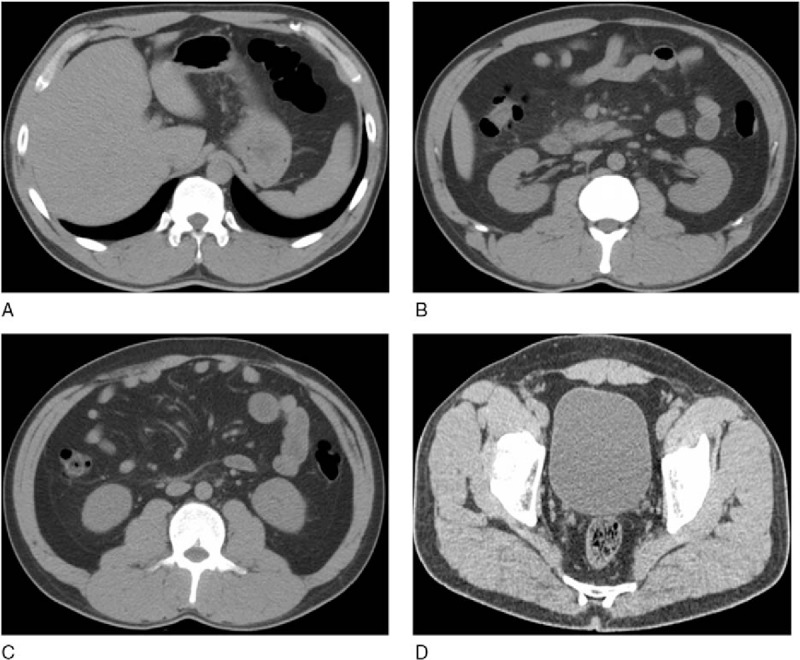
Transverse unenhanced abdominal CT scans of a 41-year-old man with AP who was discharged from the hospital 6 days after admission and had an EPIC score of 1. (A) Pleural effusions not present. (B) Right retroperitoneal inflammation. (C and D) Mesenteric inflammation and pelvic ascites not present. AP = acute pancreatitis, CT = computed tomography, EPIC = extrapancreatic inflammation on computed tomography.
